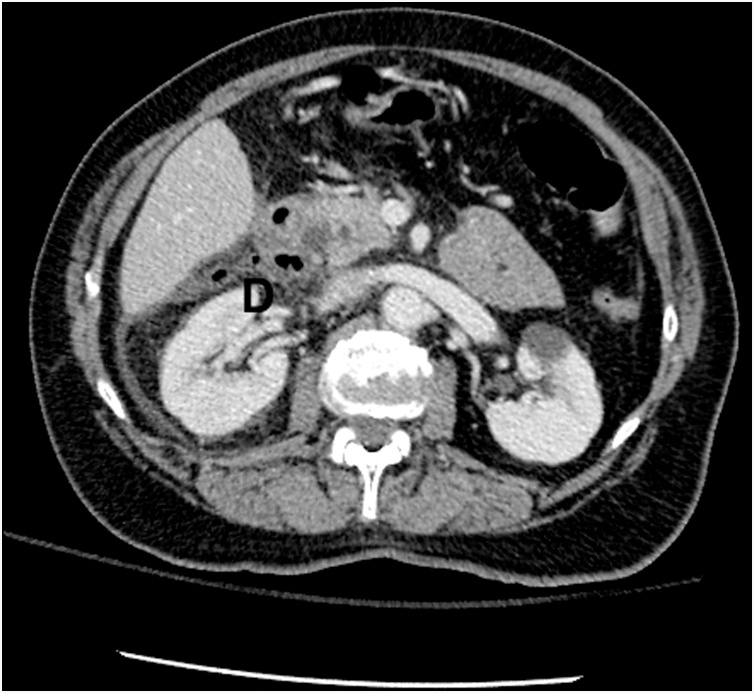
CT scan of perforated duodenal diverticulum marked with letter D.
